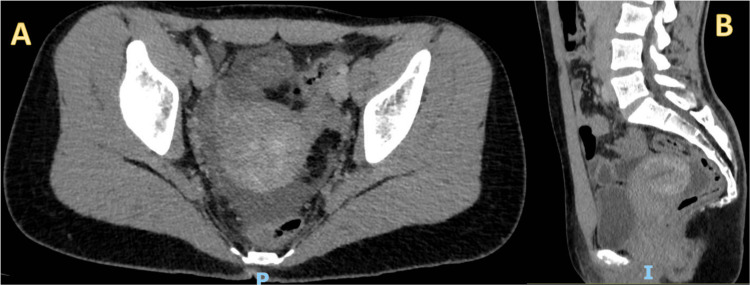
A: Axial view of contrast abdominopelvic CT showing homogeneous free fluid in the rectouterine pouch, extending to the right iliac fossa. B shows a sagittal view.
